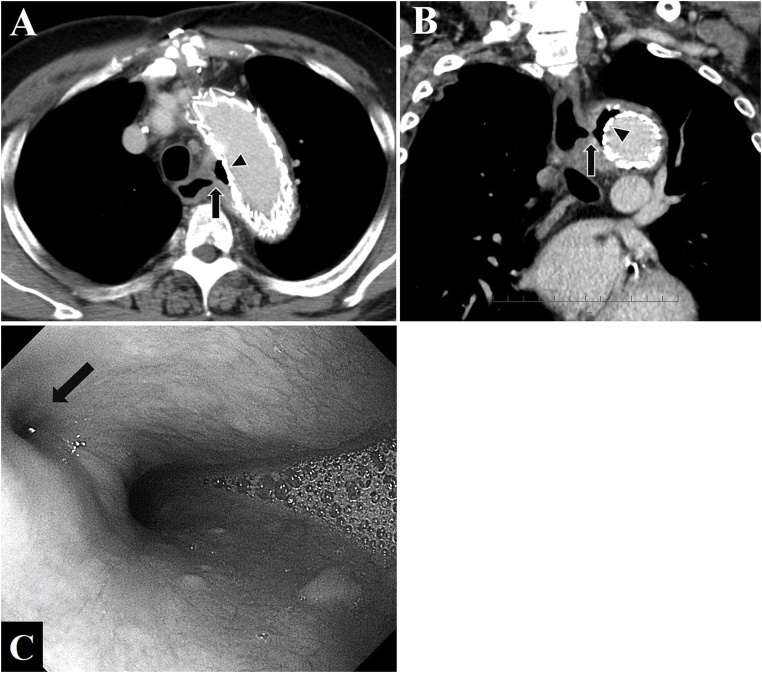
A, B. Computed tomography images of fistula between aorta and esophagus (arrow) and free air surrounding aortic stent-graft (arrowhead). A, axial image. B, coronal image. C. Upper gastrointestinal endoscopy shows ulcer on left lateral wall of upper thoracic esophagus (arrow).
